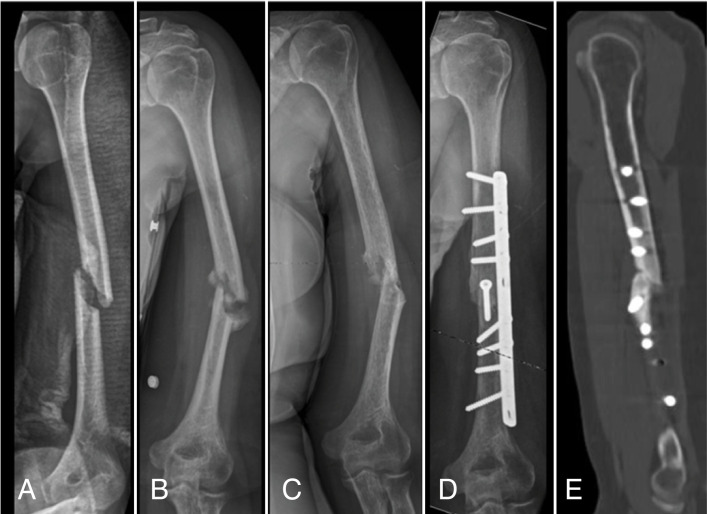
Images of the patient 1. X-rays showing the initial aspect of the fracture, evolution of 17 months, 29 months, 41 months (A, B, C and D); and tomography after 41 months of the fracture (E).
